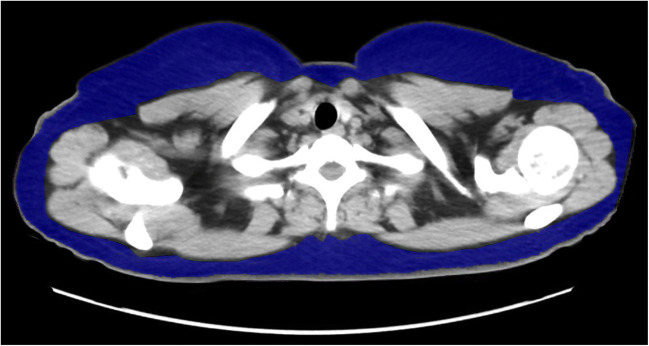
A typical image used to measure the subcutaneous fat area in the midclavicular line using multi-detector computed tomography data. Regions in blue indicate the subcutaneous fat area
